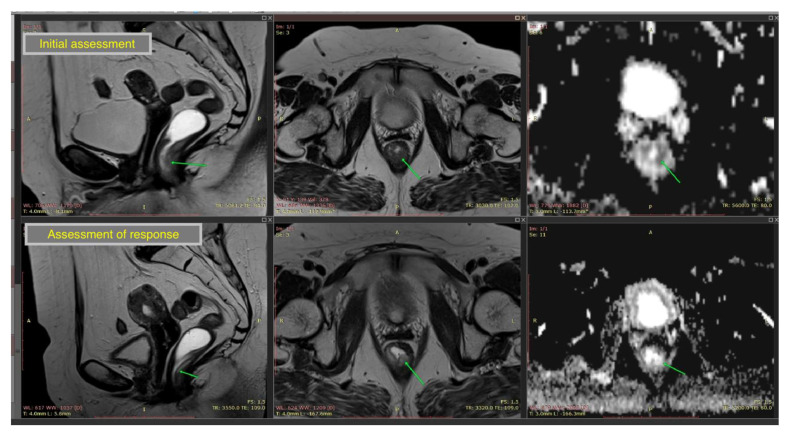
Magnetic resonance imaging initial assessment (upper three images) and response evaluation (lower three images) in an inferior rectum cancer, showing parietal thickening maintained and treatment-related changes in a patient with pathological complete response. Green arrow showing rectal tumor
